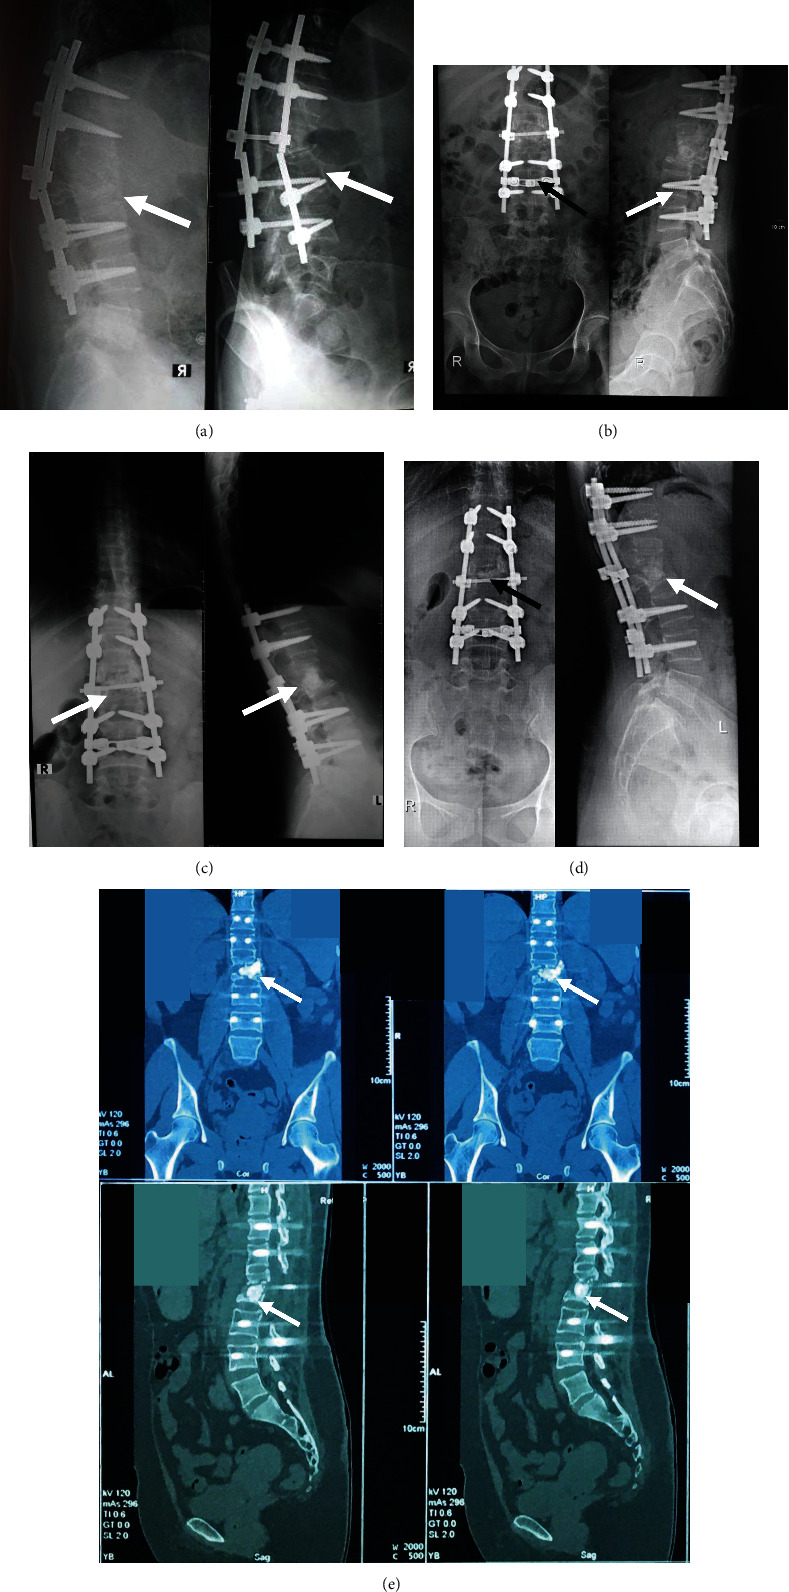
A 29-year-old female (case 1). (a) Preoperative radiography showed large destruction on the corpus vertebrae which causes nonunion and implant failure. (b) Early postoperative radiography showed implant planted posteriorly bridging defected bone with alignment well corrected. (c) Three-month postoperative radiography showed 60% of new bone growth on the defected corpus vertebrae. (d) Six-month postoperative radiography showed 80% of new bone growth on the defected corpus vertebrae. (e) Six-month postoperative CT scan showed 80% of new bone growth filling the bone defect especially on the right posterior lateral. Sites are shown using white and black arrows.
